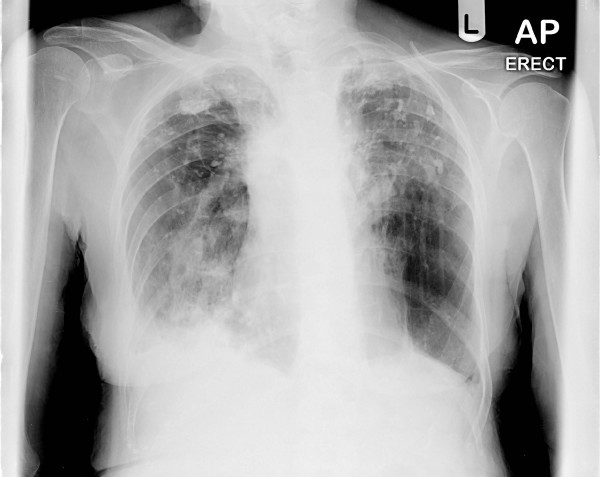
Chest X-ray showing consolidation and bronchiectatic changes.
